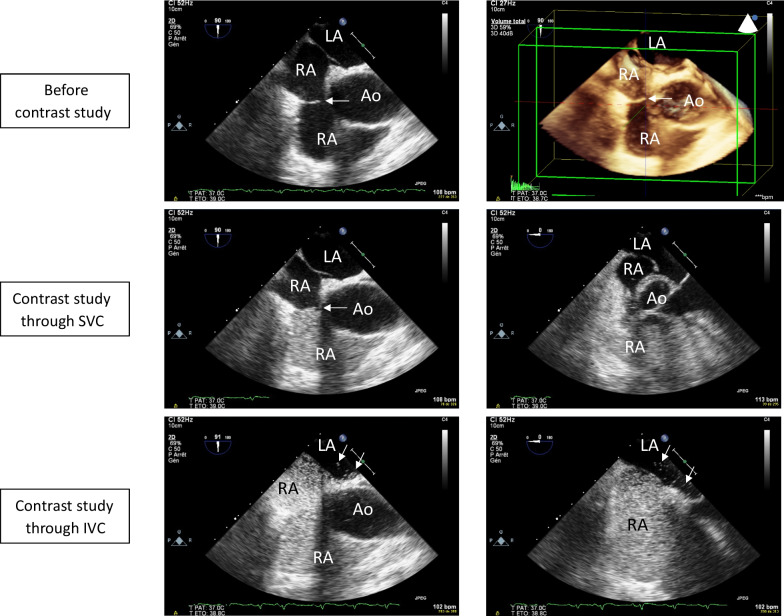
Transesophageal contrast study conducted in a ventilated ARDS patient with a prominent Eustachian valve and a *patent foramen ovale* with grade 2 interatrial right-to-left shunt. The bicaval view depicts an Eustachian valve in both two- and real-time three-dimensional imaging (upper panels, arrow). When the contrast study is performed through the superior vena cava (SVC), the microbubbles fail to reach the *fossa ovalis*, and the right atrium is incompletely opacified due to the presence of the Eustachian valve (arrow), in both the longitudinal bicaval view (90°, middle left panel) and transverse view (0°, middle right panel). When the contrast study is performed through the inferior vena cava (IVC), right atrial opacification is complete and allows the identification of a *patent foramen ovale* with microbubbles entering the left atrium during the first three cardiac cycle (arrows), in both the longitudinal bicaval view (90°, lower left panel) and transverse view (0°, lower right panel). Abbreviations: *LA* left atrium, *RA* right atrium, *Ao* ascending aorta, *SVC* superior vena cava, *IVC* inferior vena cava
